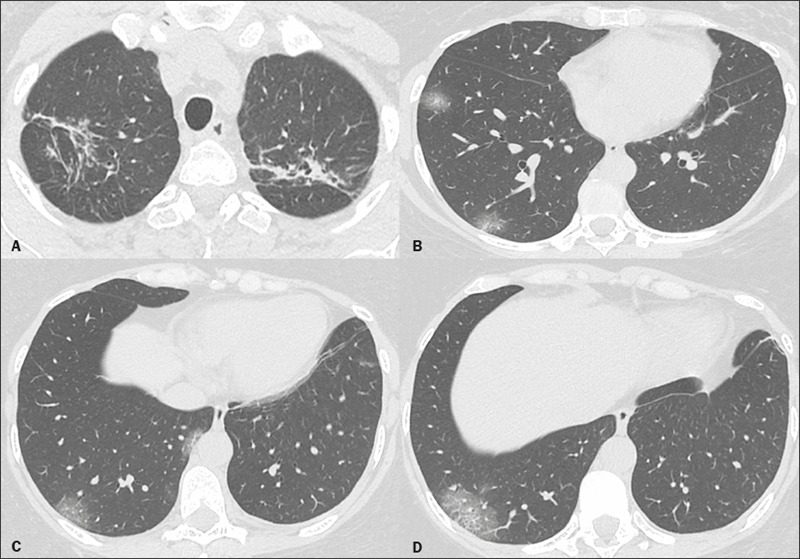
A 50-year-old woman with COVID-19 and tuberculosis. Chest CT showing
